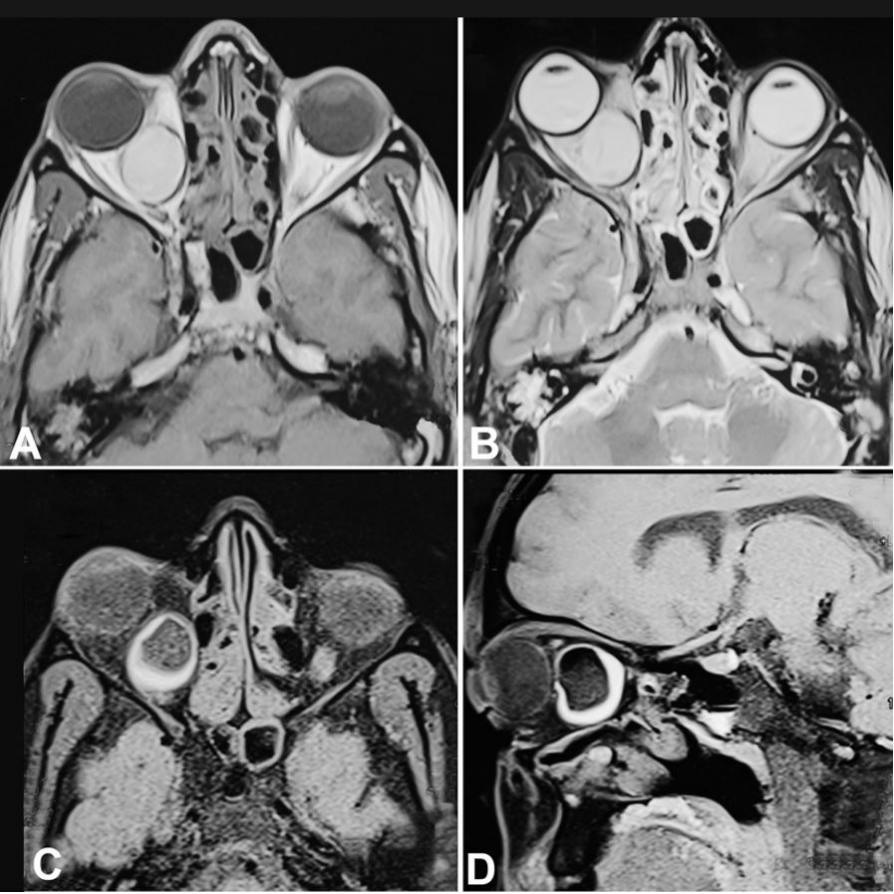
Orbit MRI. The cyst showed a hyperintense signal in both T1 (A) and T2-weighted images (B); C and D – T1- weighted image with fat-saturated sequence shows hypointense signals.
